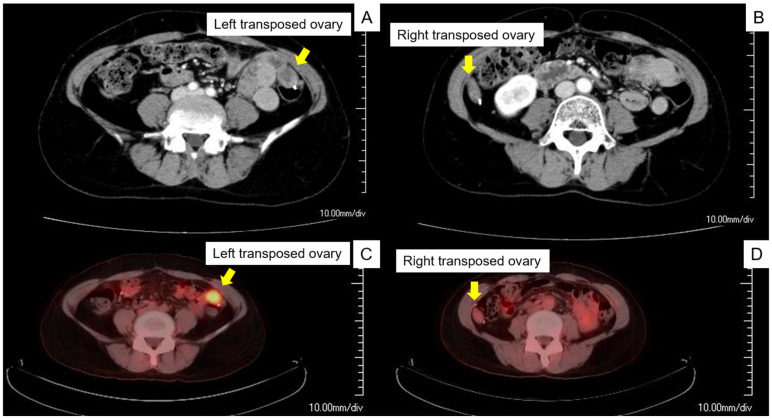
Clinical imaging of the recurrence of the transposed ovaries. (A) Contrast computed tomography (CT) scan revealed a slightly swollen left transposed ovary located in the uterine corpus. (B) The right ovary was not swollen on the contrast CT scan. (C,D) PET-CT revealed increased 18F-fluorodeoxyglucose uptake in the left transposed ovary and no increase in the right ovary. Yellow arrows indicate the left and right ovaries, respectively. Abbreviation: PET-CT, positron emission tomography–computed tomography.
